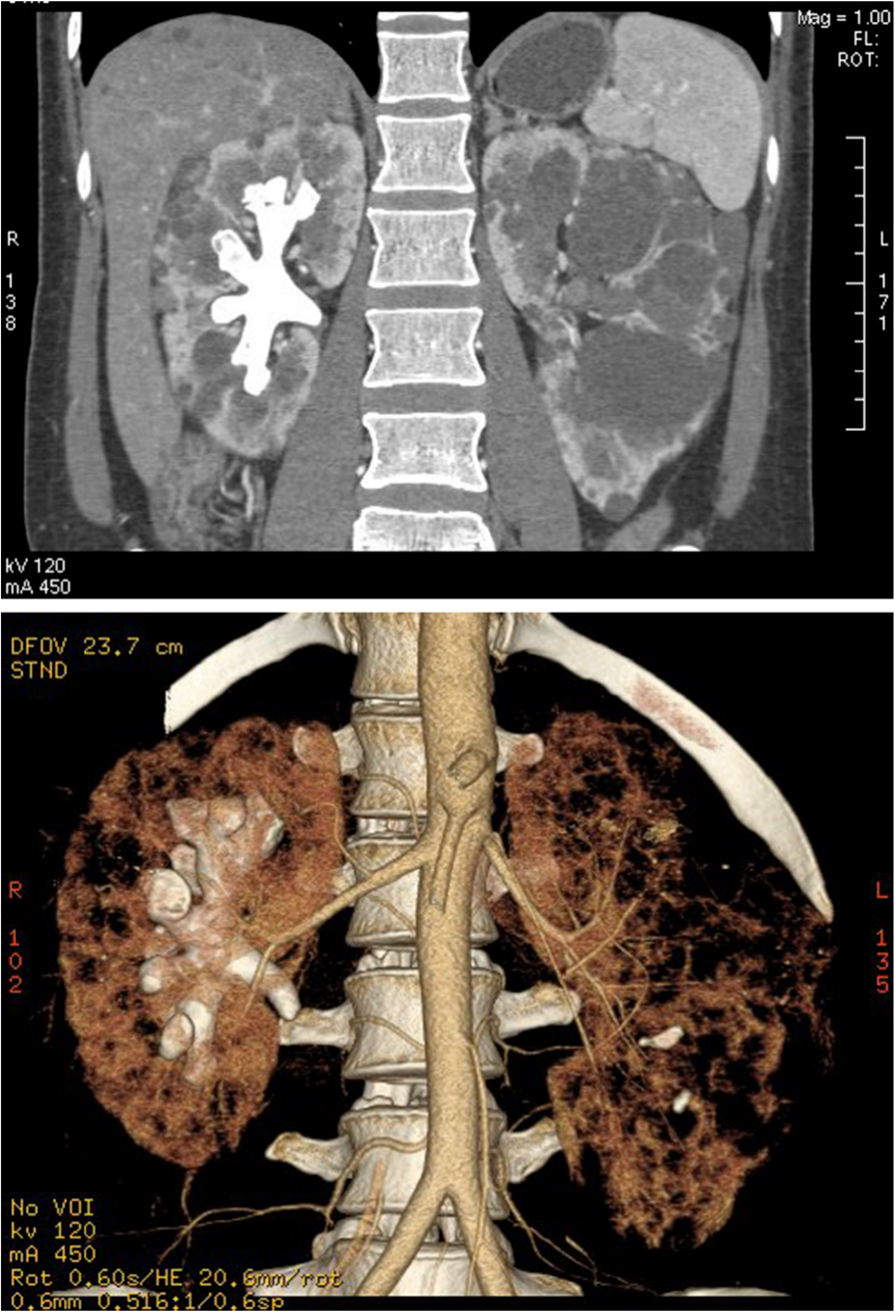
CT images of a complete staghorn calculus in the right kidney in an ADPKD female. Above the staghorn calculus and polycystic kidney in the coronal plane; Below the three-dimension reconstructed CT image of calculus and kidney.
